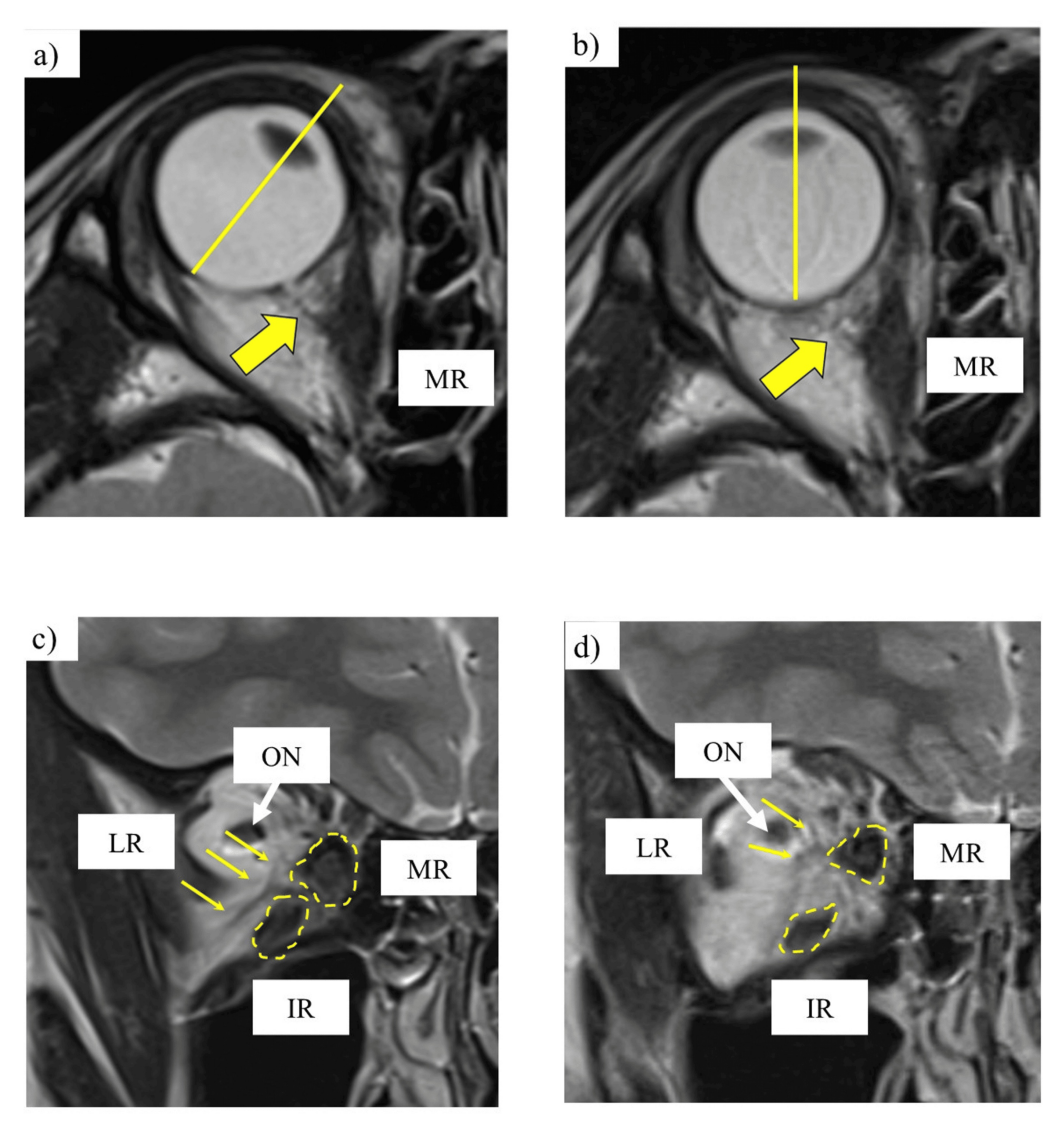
Pre- and postoperative MRIs of the orbit (a, b) Axial T2-weighted MRIs before and after surgery. (c, d) Coronal T2-weighted MRIs before and after surgery. Postoperatively, the accessory extraocular muscle (yellow arrows) previously attached to the posterior aspect of the globe from the MR appeared relaxed (a, b), thinner, and displaced upward. The bellies of the MR and IR (yellow dot circles) also appeared thinner (c, d). All images are 3.0-mm thick. LR: lateral rectus muscle, ON: optic nerve, MR: medial rectus muscle, IR: inferior rectus muscle, MRI: magnetic resonance imaging
